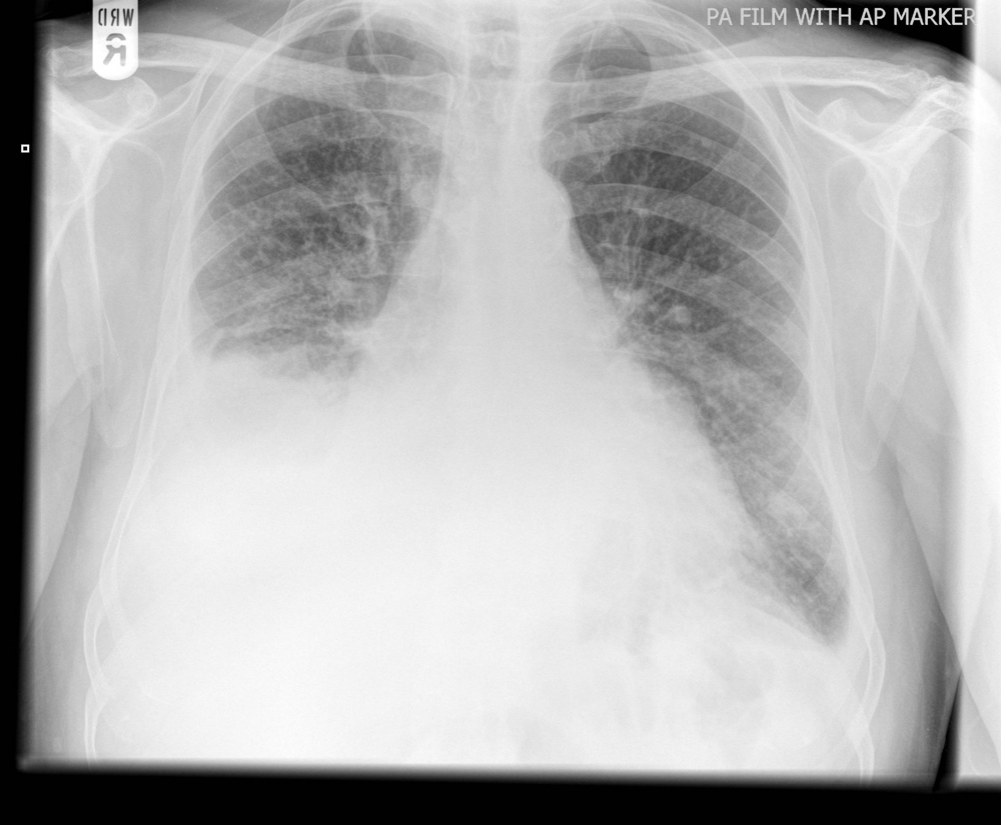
An AP view chest radiograph showing right-sided pleural effusion and right basal consolidation.
